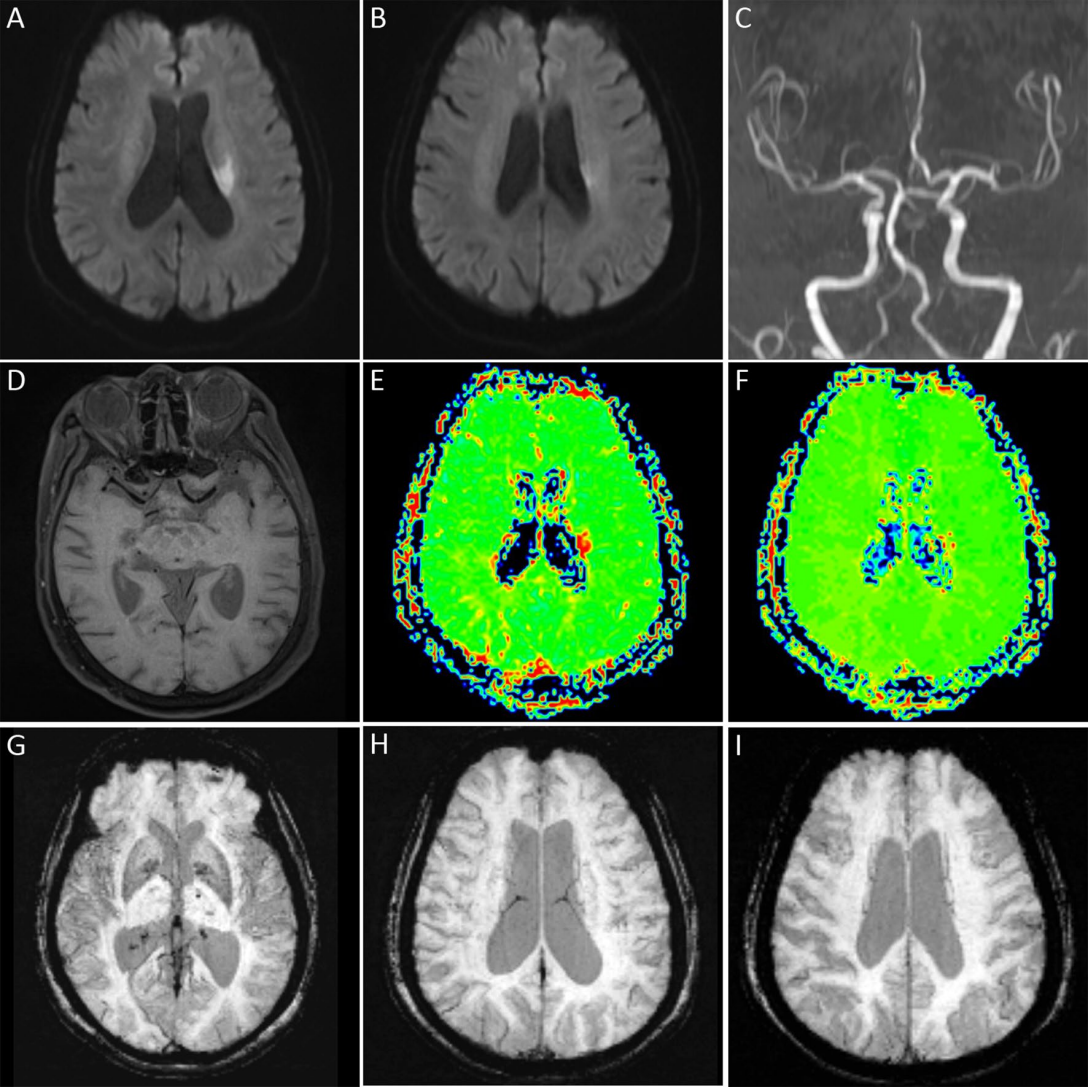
Prominent vessel sign was not displayed in a patient with perforator occlusion in the anterior circulation. DWI (A,B) showed lacunar infarction in the left paraventricular region. Large vessel occlusion was not seen on MRA (C) and 3D-SPACE (D). The MTT (E) and TTP (F) maps indicated a small area of hypoperfusion near the left lateral ventricle. There was no prominent vessel sign on SWI (G–I).
